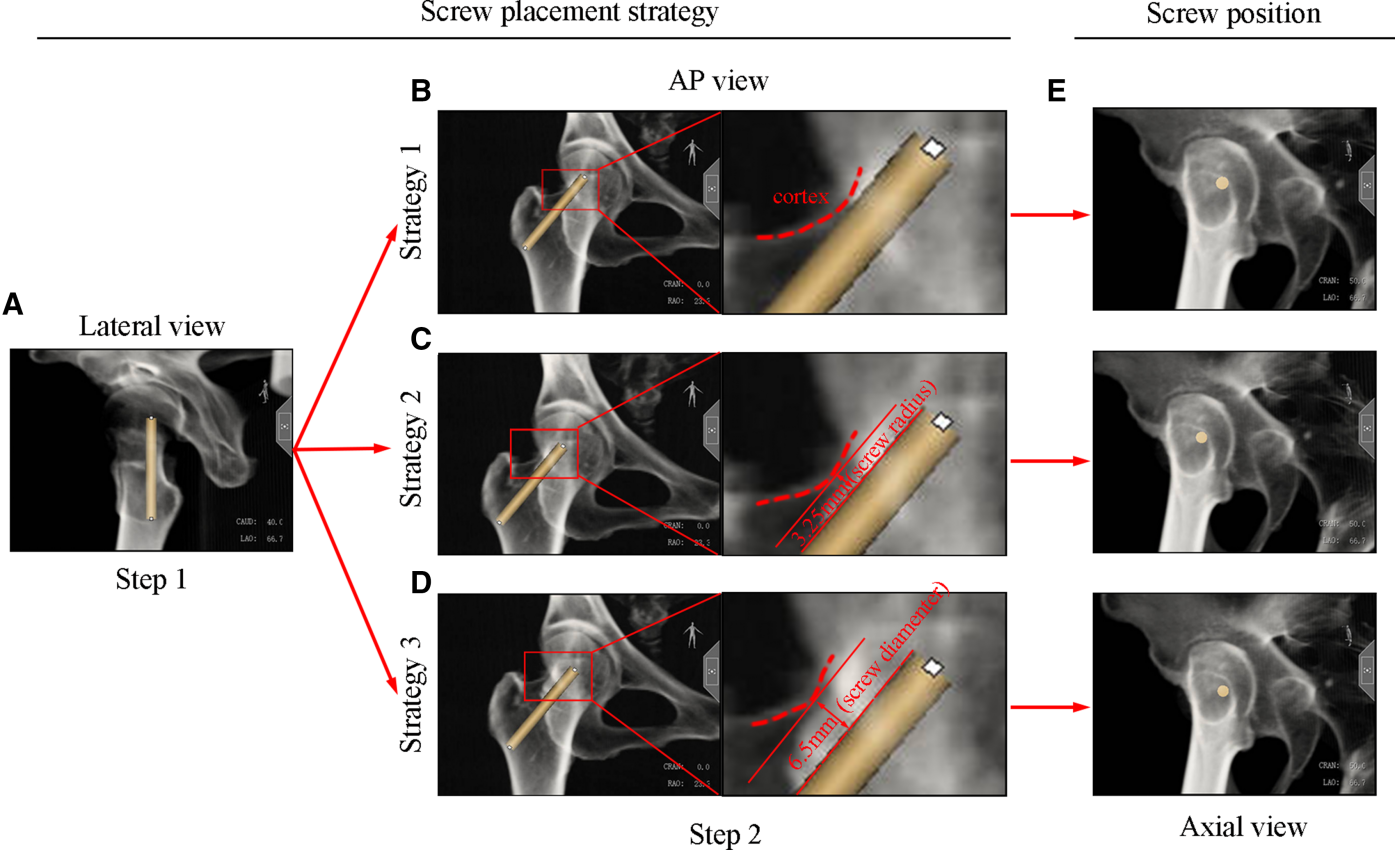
Three screw placement strategies and corresponding axis radiographs.
